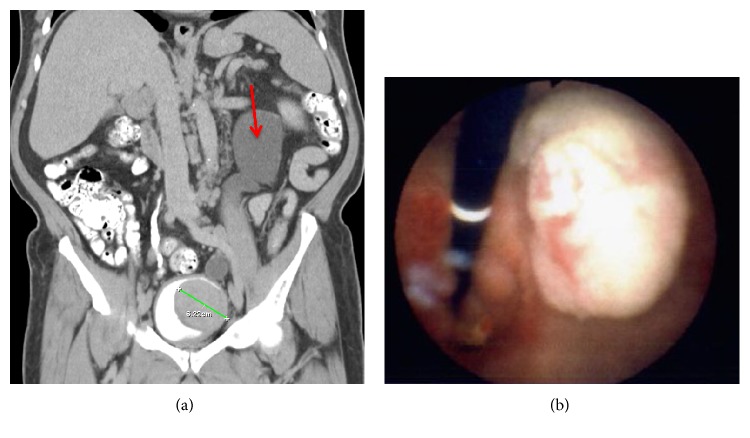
(a) CT Urogram, delayed phase, coronal view, showing 6 cm intravesical tumor and left sided hydroureteronephrosis (red arrow) due to mass effect at the level of the left ureteral orifice. (b) Cystourethroscopy revealed a pale white to tan exophytic bladder mass overlying the left ureteral orifice which was obscuring and obstructing the left kidney and ureter.
